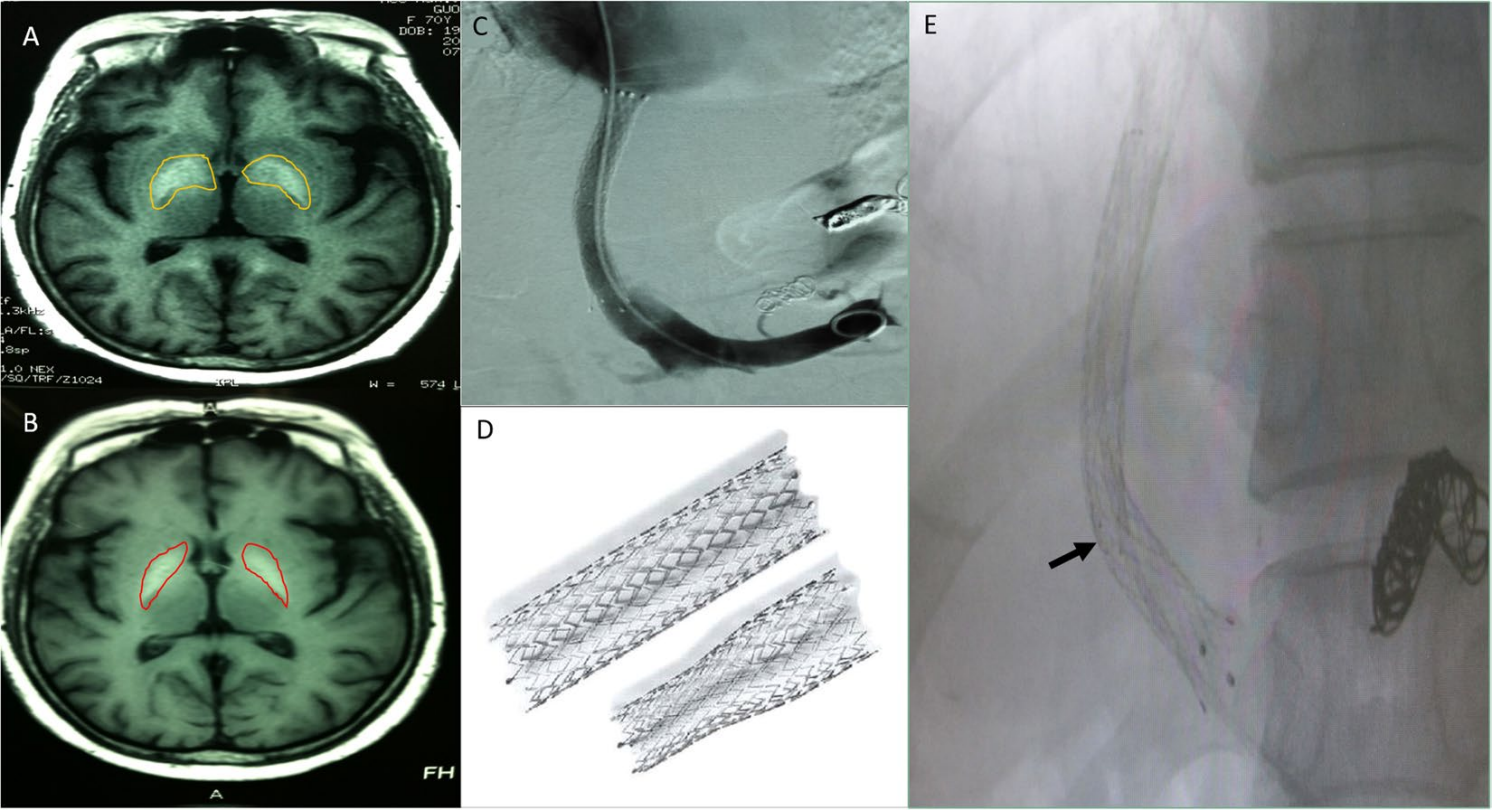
TIPS shunt limiting and cranial MRI changes. (A) Before stent limiting therapy, the cranial MRI T1WI exhibited high signals in the globus pallidus of the basal ganglia (yellow area). (B) After local stent-limiting about three months, the cranial T1WI MRI identified no aggravation and a slight decrease in the signals of basal ganglia (red area). (C) Angiography of portal vein after TIPS showed unobstructed stent and no abnormal varicose vein. The diameter of original shunt was 10 mm. (D) Normal straight stent and tapered stent image (below is 7–10 × 40 mm, ev3 Protégé Rx taper stent). (E) Patients underwent placement of a 7–10 mm (diameter) stent inside the original 10 mm stent. The distal point of stent was highlighted with arrows.
